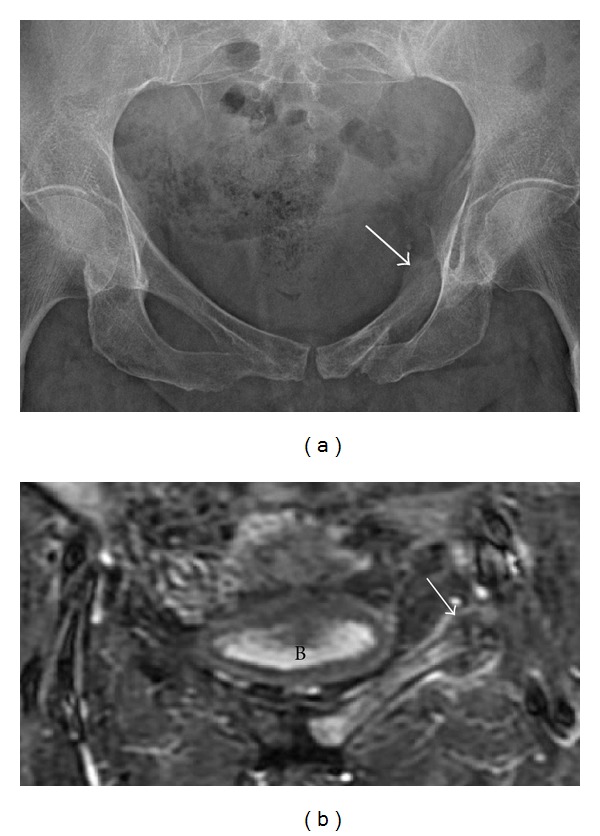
Insufficiency fracture. A 40-year-old male with a pelvic insufficiency fracture. Radiograph (a) demonstrates an expansile osseous lesion with periosteal reaction and maturing callus along the left superior pubic ramus (arrow). No definite fracture line is identified. Coronal STIR (b) of the pelvis demonstrates bone marrow edema throughout the left superior pubic ramus, fracture site, and surrounding callus (arrow). B: bladder.
